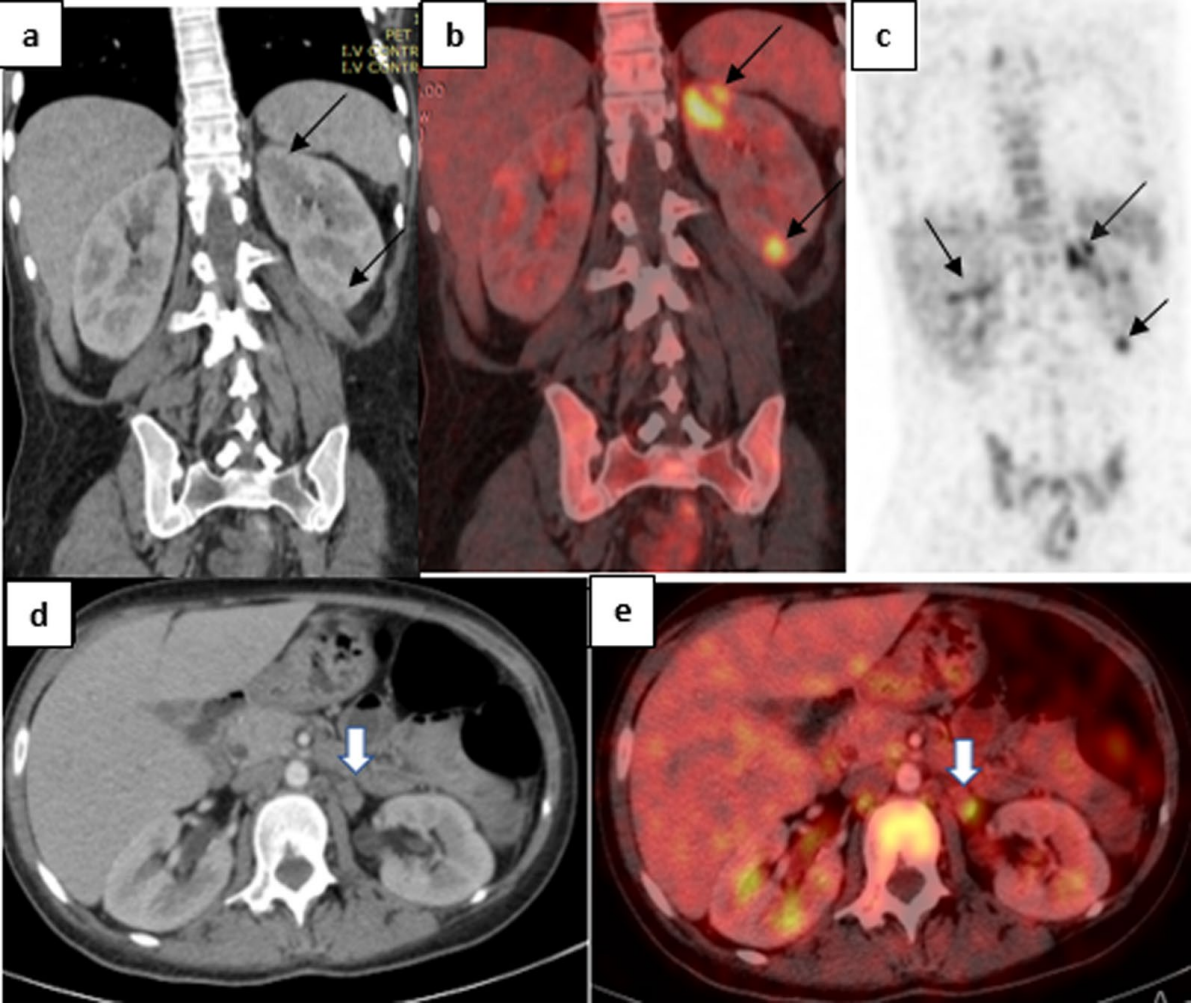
36-year-old female with history of SLE (systemic lupus erythromatosis) presented with fever of unknown origin. a Coronal CT image. b Coronal Fused PET/CT image and c Coronal PET image of the upper abdomen showing bilateral renal cortical hypodense metabolically active lesions (larger, more in number and more avid on the left side) (black arrows). d, e are axial CT and Fused PET/CT of the abdomen at the same level showing enlarged low metabolically active regional lymph nodes. The patient was diagnosed as lupus nephritis and responded to adequate treatment with appropriate doses of steroids
